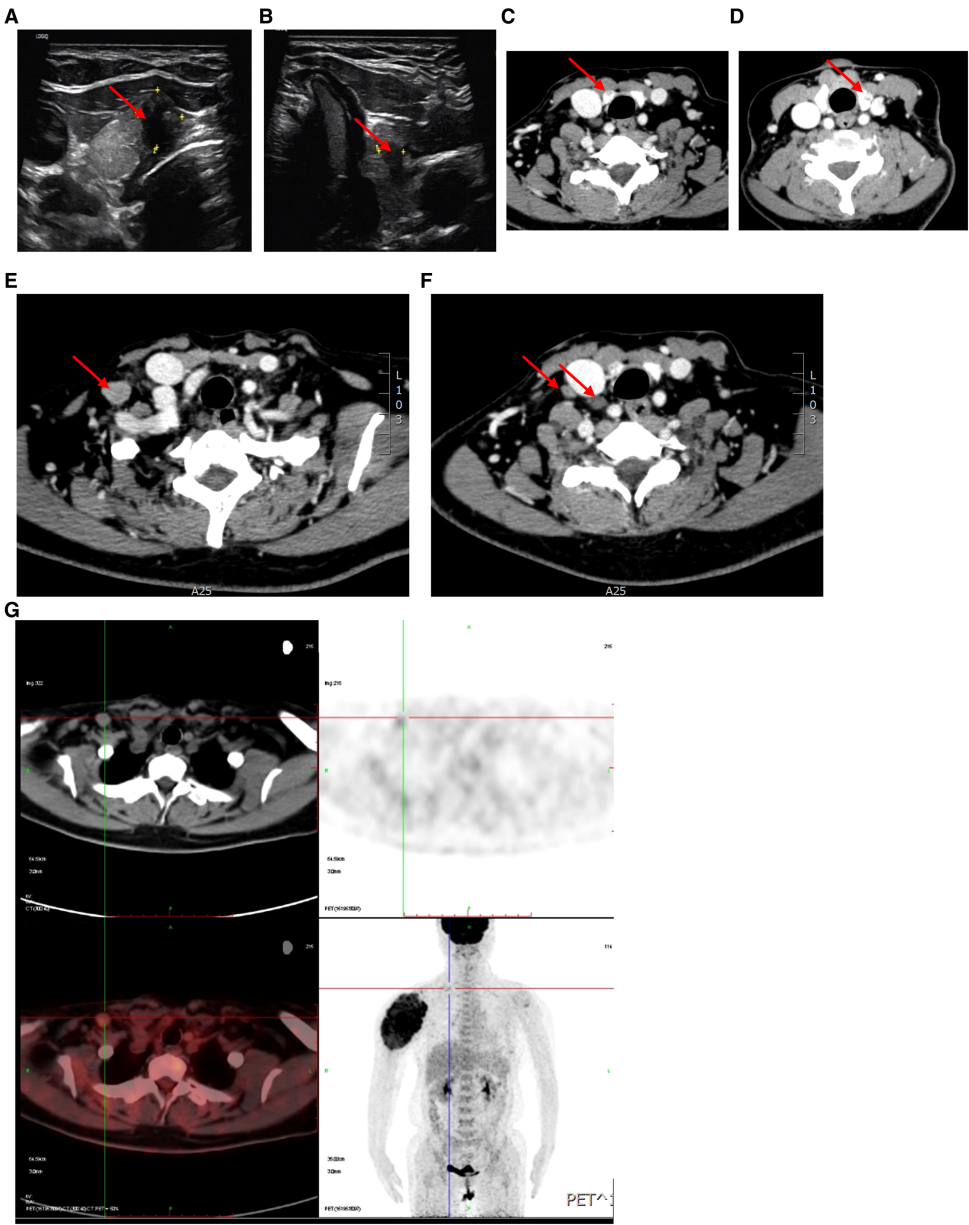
Examination of thyroid and cervical lymph nodes. (A,B) CE-US of thyroid showed within the upper portion of the left thyroid lobe was a hypoechoic, taller than wide, irregular nodule (red arrow), deeming it a TI-RADS 5. (C,D) Contrast-enhanced CT of the thyroid identified hypodense nodules within both thyroid lobes. (E,F) CT scan showed high density nodules in the right lateral cervical region. (G) ^18^F-FDG PET/CT images showed increased uptake in right supraclavicular fossa.
